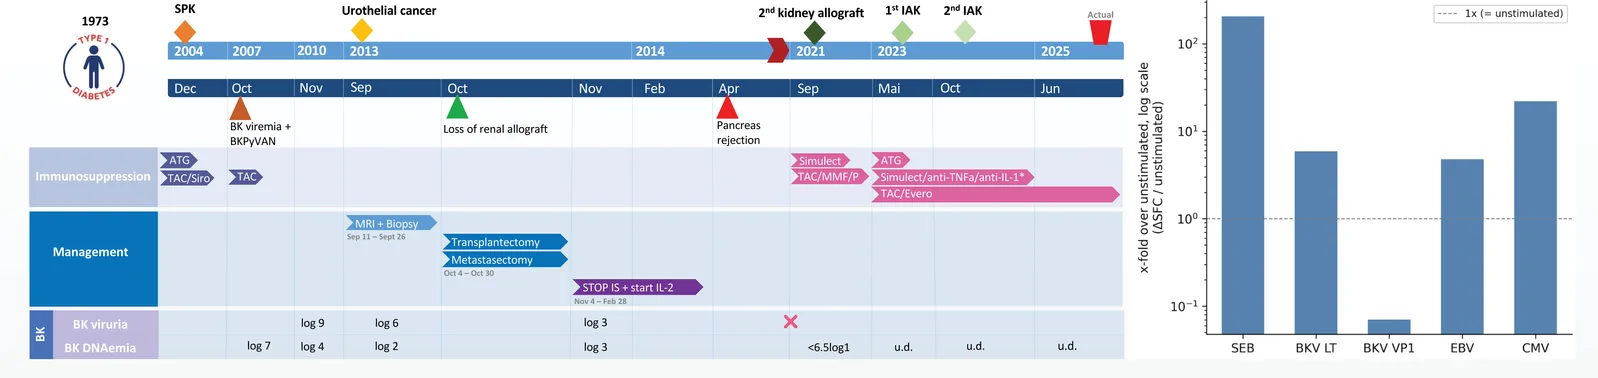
Transplantation history, clinical management and virological results Left panel: Timeline overview; right panel: BKPyV-specific cellular immune responses Abbreviations: anti-lL-1, anti-interleukin 1; anti-TNFa, anti-tumor necrosis factor alpha; ATG, thymoglobulines; BK, BK Polyomavirus; BKPyVAN, BK-associated nephropathy; Evero, everolimus; IAK, islet after kidney transplantation; IL-2, interleukine-2; IS, immunosuppression; MMF, mycophenolate mofetil; MRI, magnetic resonance imagery; P, prednisone; Siro, sirolimus; SPK, simultaneous pancreas-kidney transplantation; TAC, tacrolimus . BK viruria is expressed in log10 (copy/ml); BK DNAemia is expressed in log10 (copy/ml until 2022, then in IU/ml). u.d. undetectable. *Induction immunosuppression according to Edmonton protocol for both IAK. BKPyV-LTag covers the viral tumor antigen 15mer peptides, whereas BKPyV-Vp1 15mer peptides covers the capsid antigen. IFN-γ response expressed as ΔSFC (spot forming cells per million PBMCs, ΔSFC/10^6^ cells). ΔSFC represents the net BKPyV-specific response after background substraction, accounting for baseline T-cell activation and nonspecific IFN-γ secretion observed in unstimulated conditions. Unstimulated wells reflect baseline T cell activity irrespective of antigen specificity. Assay controls were adequate, with a negative control of 23.3 SFC/10^6^ PBMCs and a strong response to Staphylococcal Enterotoxin B (SEB; Δ 4,887 SFC/10^6^ PBMCs). SEB served as a positive control for nonspecific T cell activation. EBV was assessed using consensus 15mer peptide pool and CMV using pp65 15mer peptide pools.
